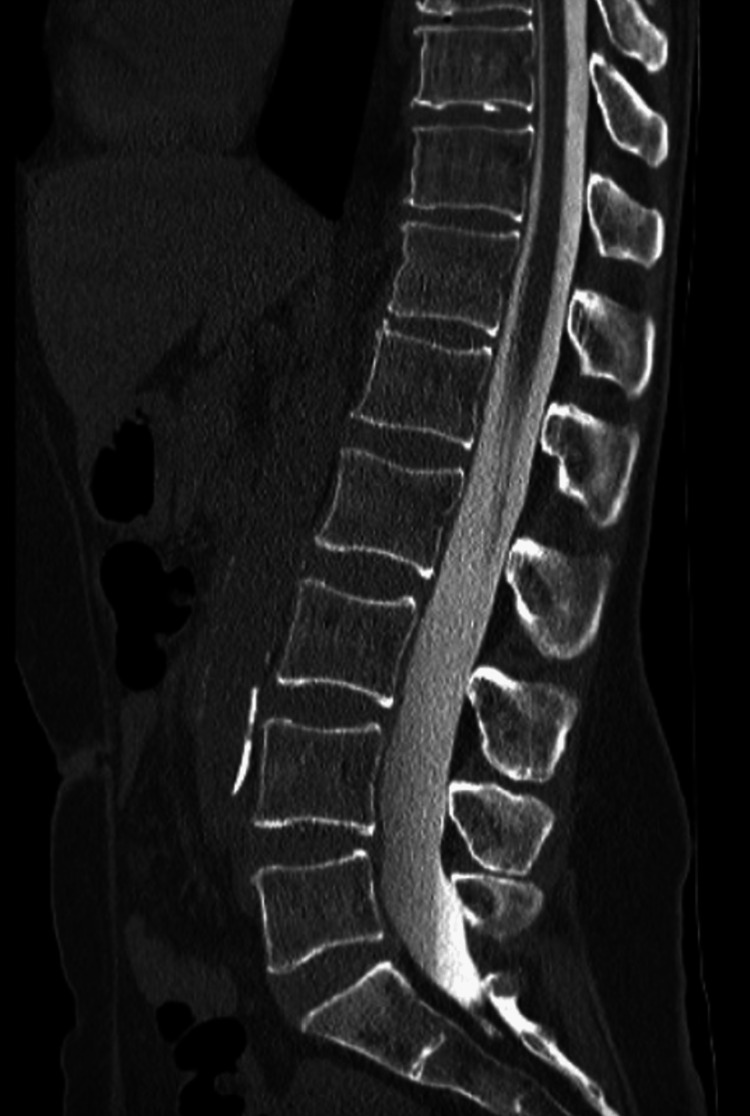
CT myelogram of the lumbar spine performed 20 days following her previous brain CT demonstrates excellent opacification of the thecal sac with contrast and no evidence of a CSF leak or subdural collections.
